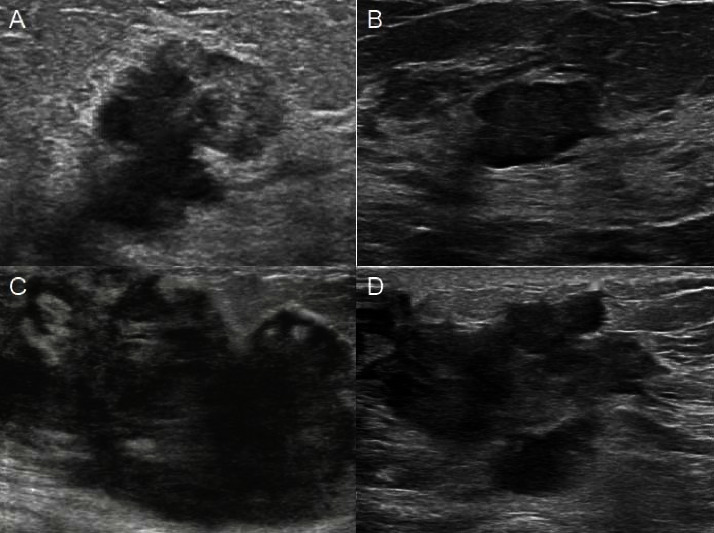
Ultrasound images of two patients who received NAC and belonged to the MHR group. (a, c) Images before NAC. (b, d) Images after NAC ends.
